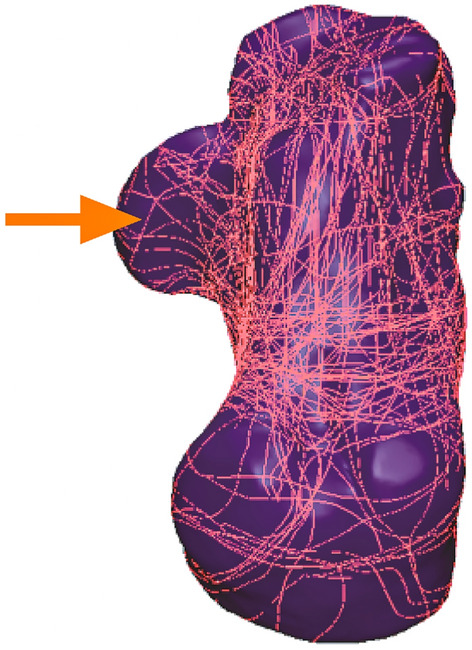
The method used for 3D CT mapping of sustentacular fracture. Representative views of the 3D map of the 81 calcaneal fracture lines.
